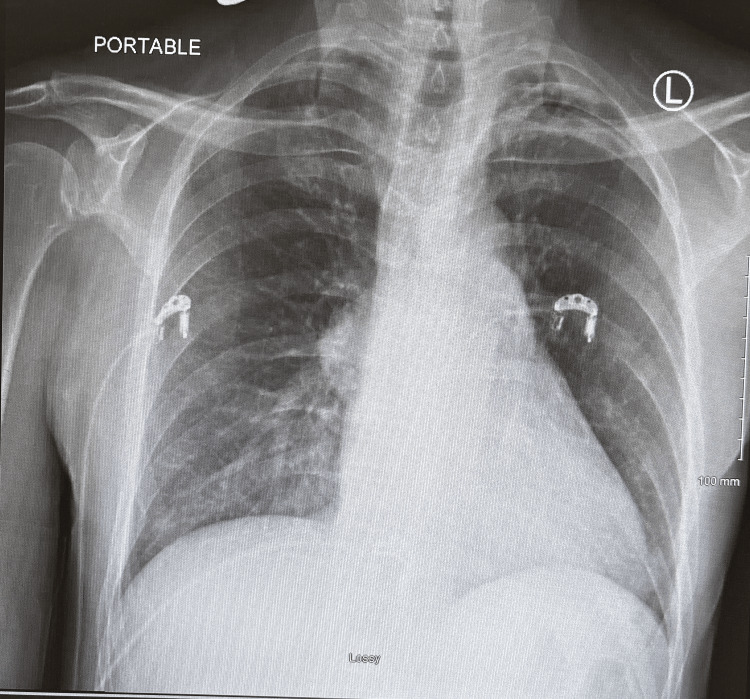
Chest X-ray from admission showing no acute cardiopulmonary pathology
